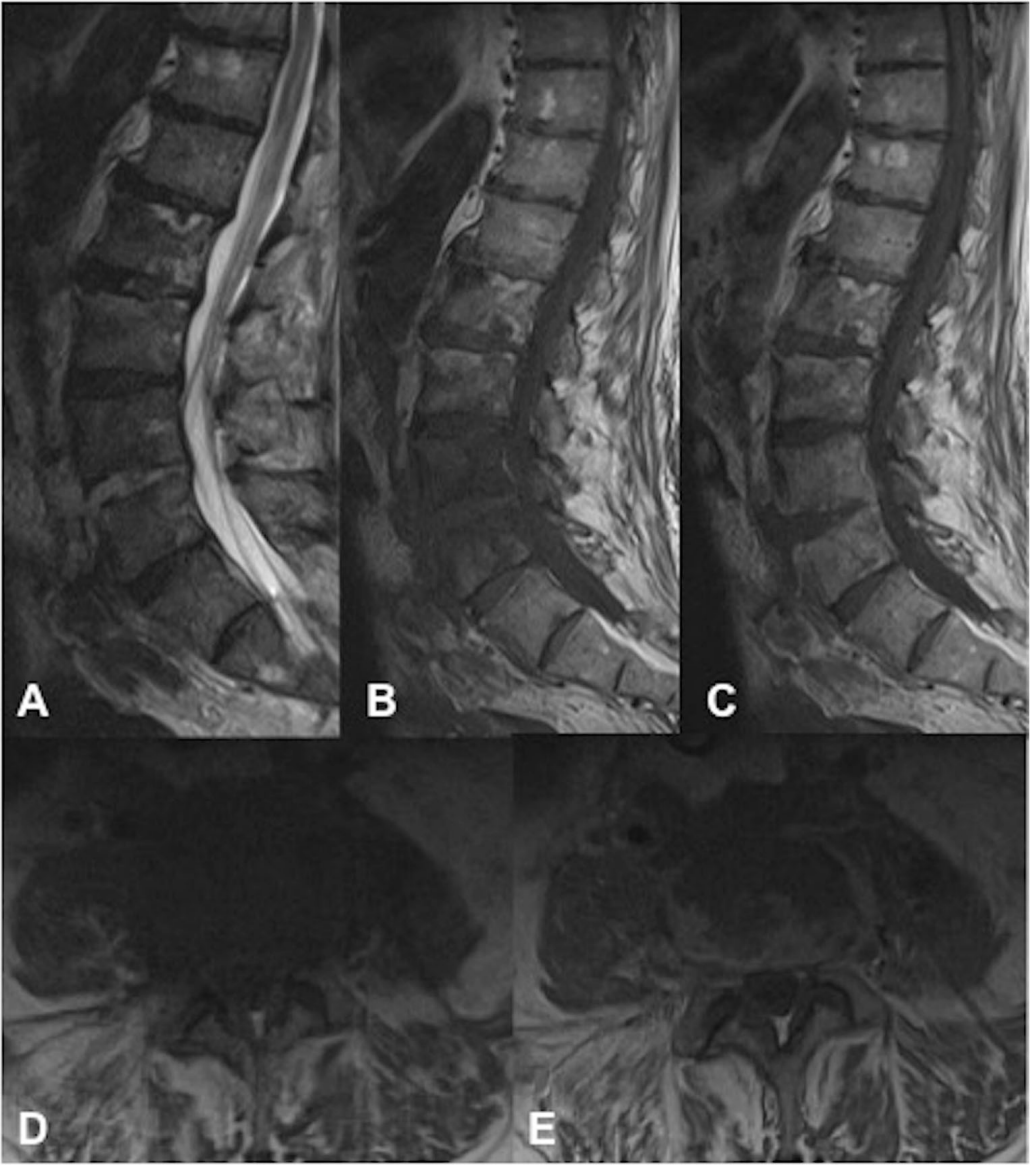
A 70-year-old man (patient 3). a Sagittal T2, b T1 pre-contrast and c T1 post-contrast sequences demonstrating increased T2-weighted signal centred on the L4/5 disc with anterior paravertebral extension corresponding to a peripherally enhancing abscess. d Axial T1 pre-contrast and e T1 post-intravenous contrast medium at the L4/5 disc level show contiguous peripherally enhancing disc/anterior paravertebral abscess. Note the lack of extension posterior to the epidural space
